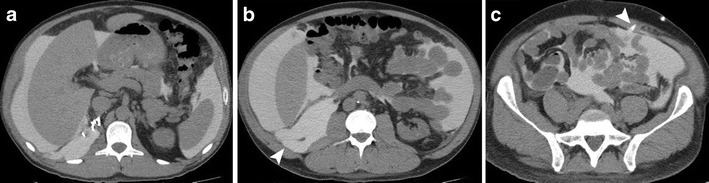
Axial CT peritoneography in a patient with prior right nephrectomy demonstrating peritoneal distribution of dialysate mixed with iodinated contrast in the upper abdomen (a) communicating into the lesser sac anterior to the pancreas. At a more inferior level in the mid-abdomen (b) with dialysate seen within the right retroperitoneum at the nephrectomy bed (*arrowhead*) as a consequence of prior surgery, and in the pelvis (c) surrounding large and small bowel loops and within the mesentery with a Tenckhoff dialysis catheter seen entering the peritoneal cavity through the left rectus muscle (*arrowhead*)
